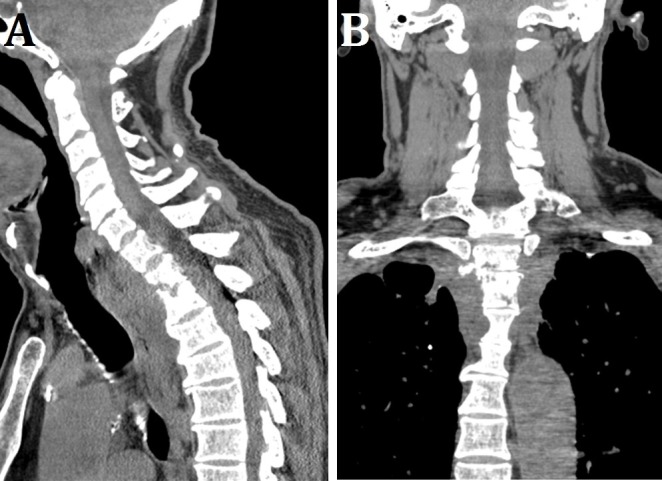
Preoperative CT Imaging ​Preoperative (A) sagittal and (B) coronal CT imaging showing erosions of the T1 and T2 vertebral bodies, with loss of the intervertebral disc space, consistent with discitis and osteomyelitis.
